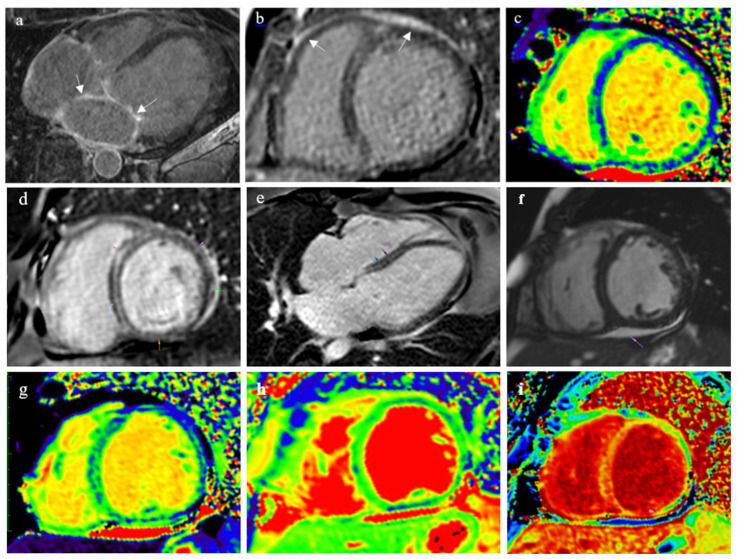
Cardiac MRI in patients with cardiomyopathy and post-COVID myocarditis. Patient A, 44-year-old man results (a–c): late gadolinium enhancement in atria ((a)—4-chamber view) and ventricles subepicardially ((b)—short axis view), T1 map ((c)—short axis view, diffuse T1 changes along the posterior septal segment). Patient with laminopathy, 44-year-old man, results (d–i): intramyocardial late gadolinium enhancement throughout the entire interventricular septum at basal and mid-levels, subepicardial in posterior and anterolateral segments ((d)—short axis view, (e)—4-chamber view); (f)—short axis view, pericardial effusion due to myopericarditis; (g)—short axis view, native T1 map, increase in T1 relaxation time to a maximum of 1107 ms in the posterior septal segment (N 995 ± 32), in the anterior septal, anterolateral and posterior segments; (h)—short axis view, T2 map, increase in T2 relaxation time to a maximum of 56 ms in the posterior septal segment (N 46 ± 3), in the anterior septal and posterolateral segments; (i)—short axis view, ECV map (plotted using CMR CVI 42 software, GE Healthcare), an increase in the extracellular volume (ECV) fraction maximally along the interventricular septum to 38 ± 3%.
